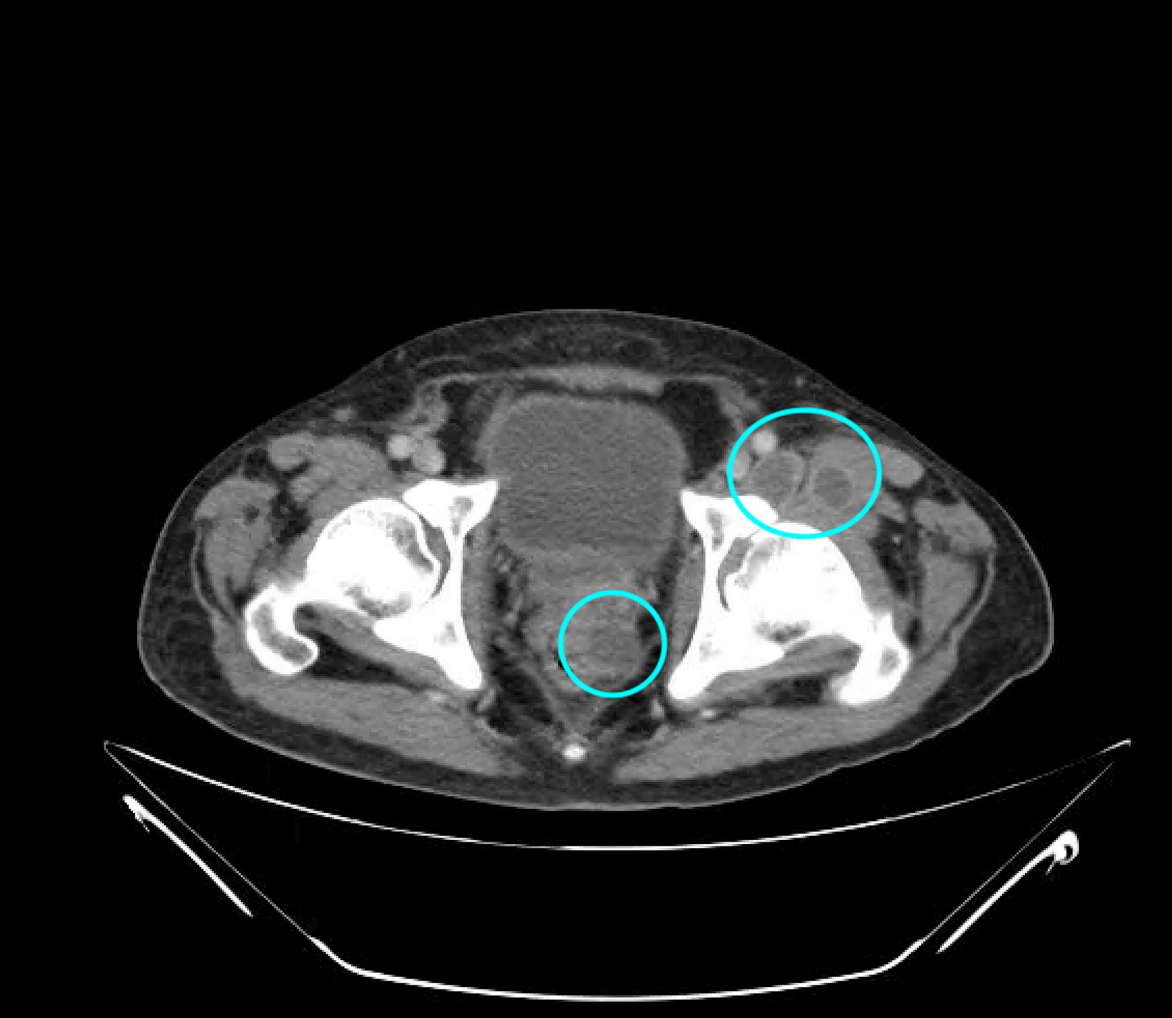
CT abdomen/pelvis showing fluid collections in the left anterior hip and perirectal area (blue circles).
